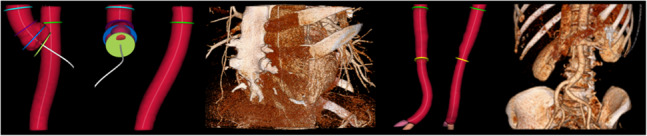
FOV of the various CTA scans. *Left: *Cardiac CTA. *Right:* Abdominal CTA. The aorta surfaces are shown from two different view points
